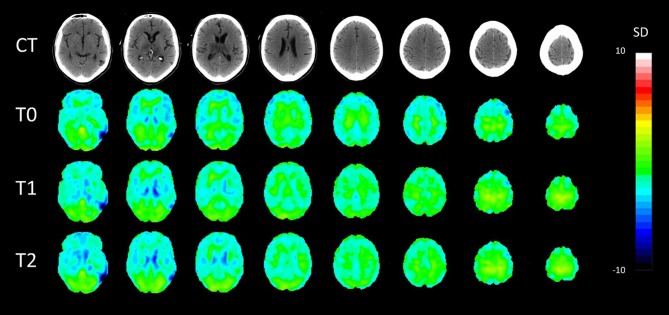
Axial patient normalization map PET with co-registered CT in the three time points. SD, standard deviation colored scale. Slices were taken with 8 mm distance.
